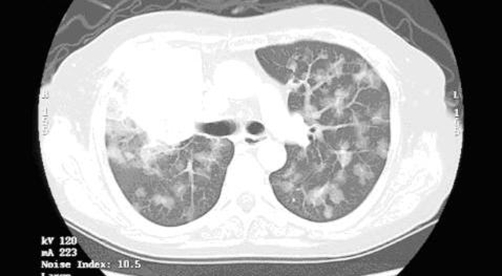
Chest CT showing a mass of the right upper lobe and multiple pure ground-glass opacity of the bilateral lungs.
